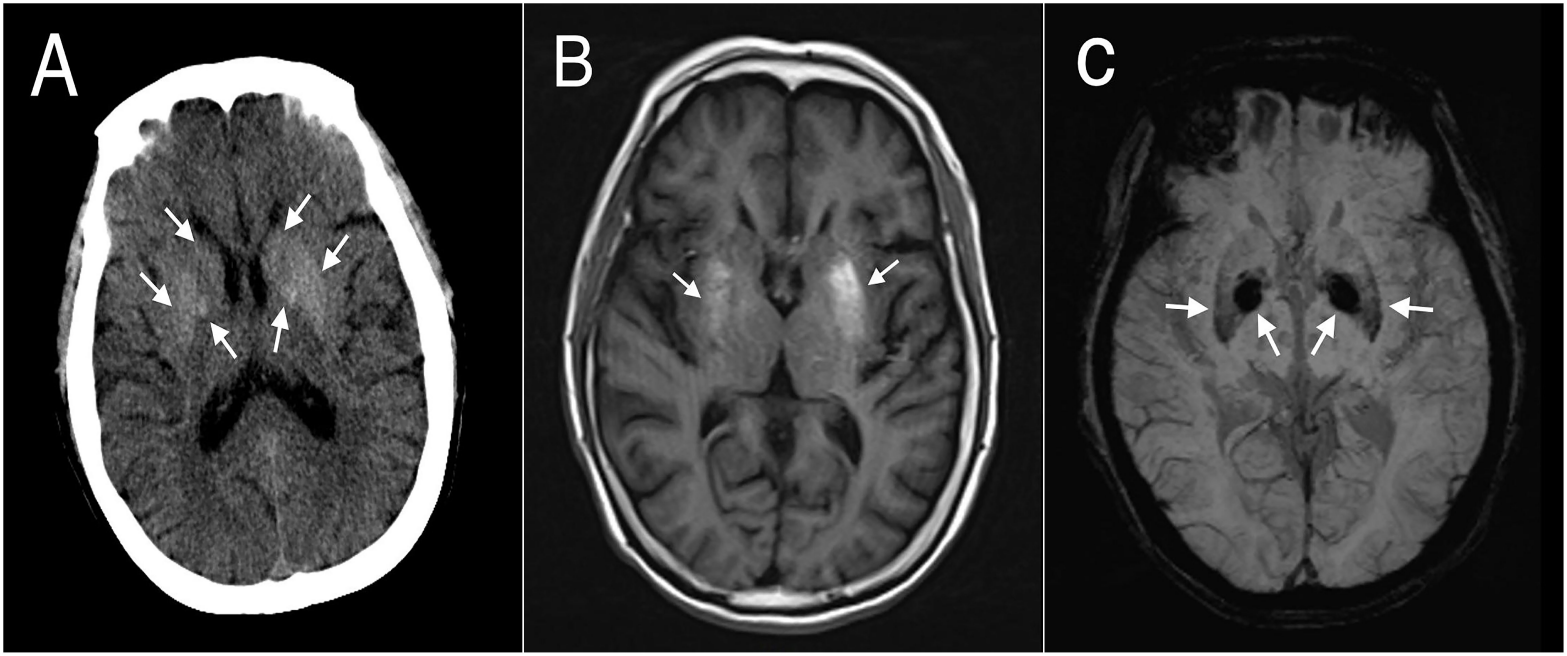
Brain computed tomography (CT) and magnetic resonance images (MRIs) of participant #7. (A) CT showed bilateral hyperdense lesions in the striatum. (B) T1-weighted MRI showed asymmetrical bilateral hyperintense lesions in the putamen, but no significant changes in the caudate or globus pallidus. (C) Susceptibility-weighted imaging showed bilateral symmetrical hypointense lesions in the putamen and globus pallidus.
